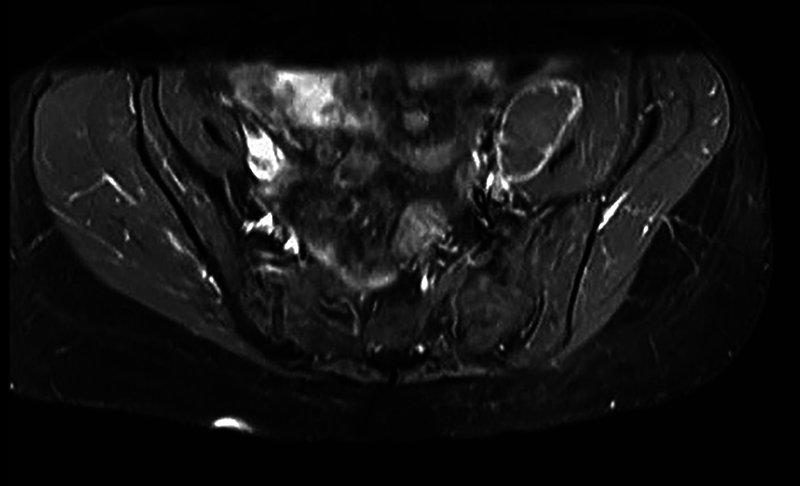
Axial T2-weighted magnetic resonance imaging (MRI) sequence of the lumbar spine, showing retroperitoneal abscess as a tuberculosis complication in patient 1.
